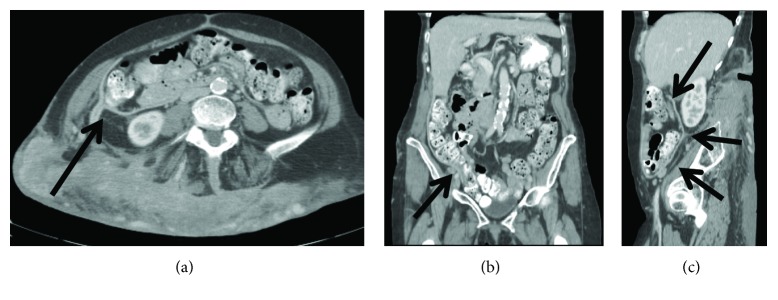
(a–c) Follow-up CT scan (3 planes) at 6 months. Hernia covered by Bio-A mesh (arrows).
